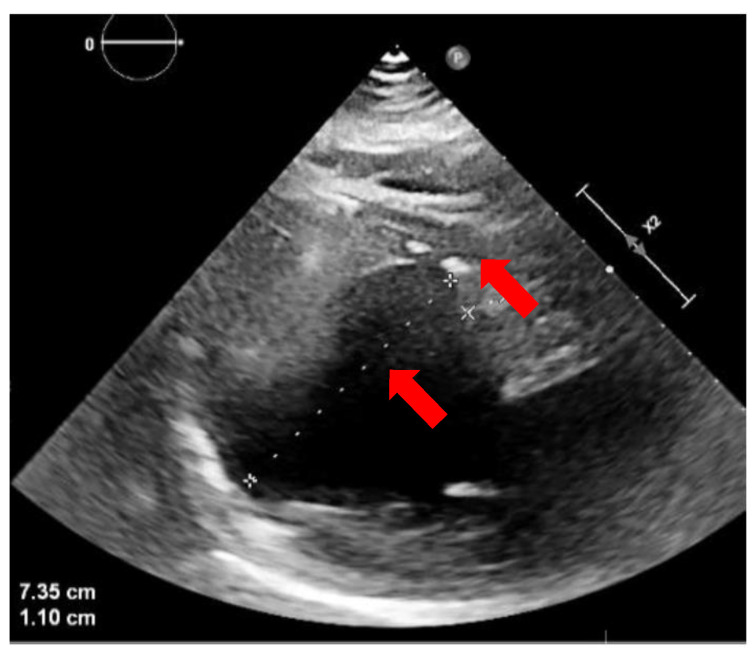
Echocardiographic image (parasternal long axis) showing a severely dilated left ventricle with apical thrombosis (red arrows).
